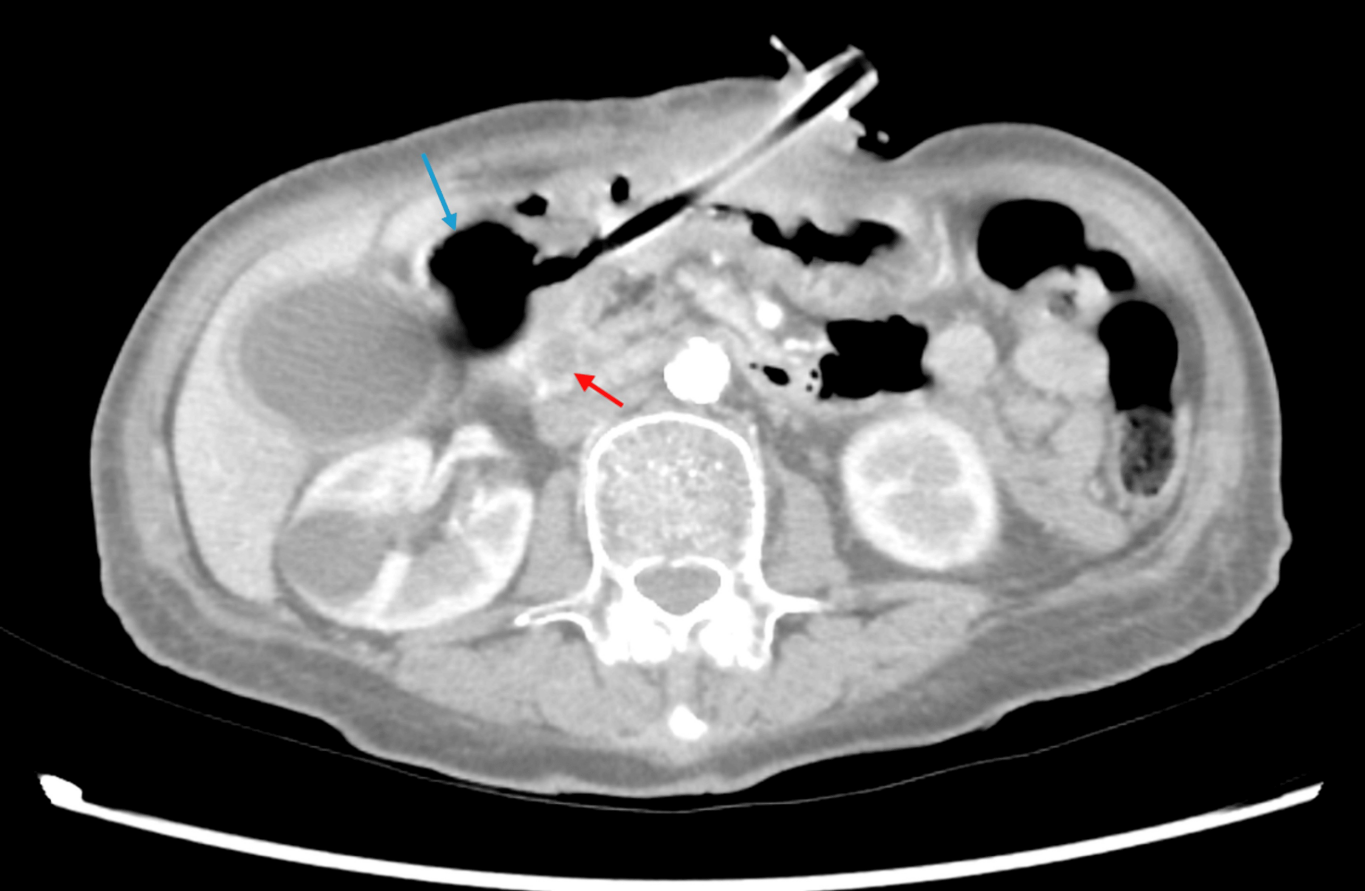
Axial computed tomography scan with intravenous contrast of the abdomen and pelvis. An axial computed tomography scan with intravenous contrast of the abdomen and pelvis demonstrated a common bile duct dilated to 11.6 mm (red arrow), secondary to mass effect from a migrated gastrostomy tube balloon in the proximal duodenum (blue arrow).
